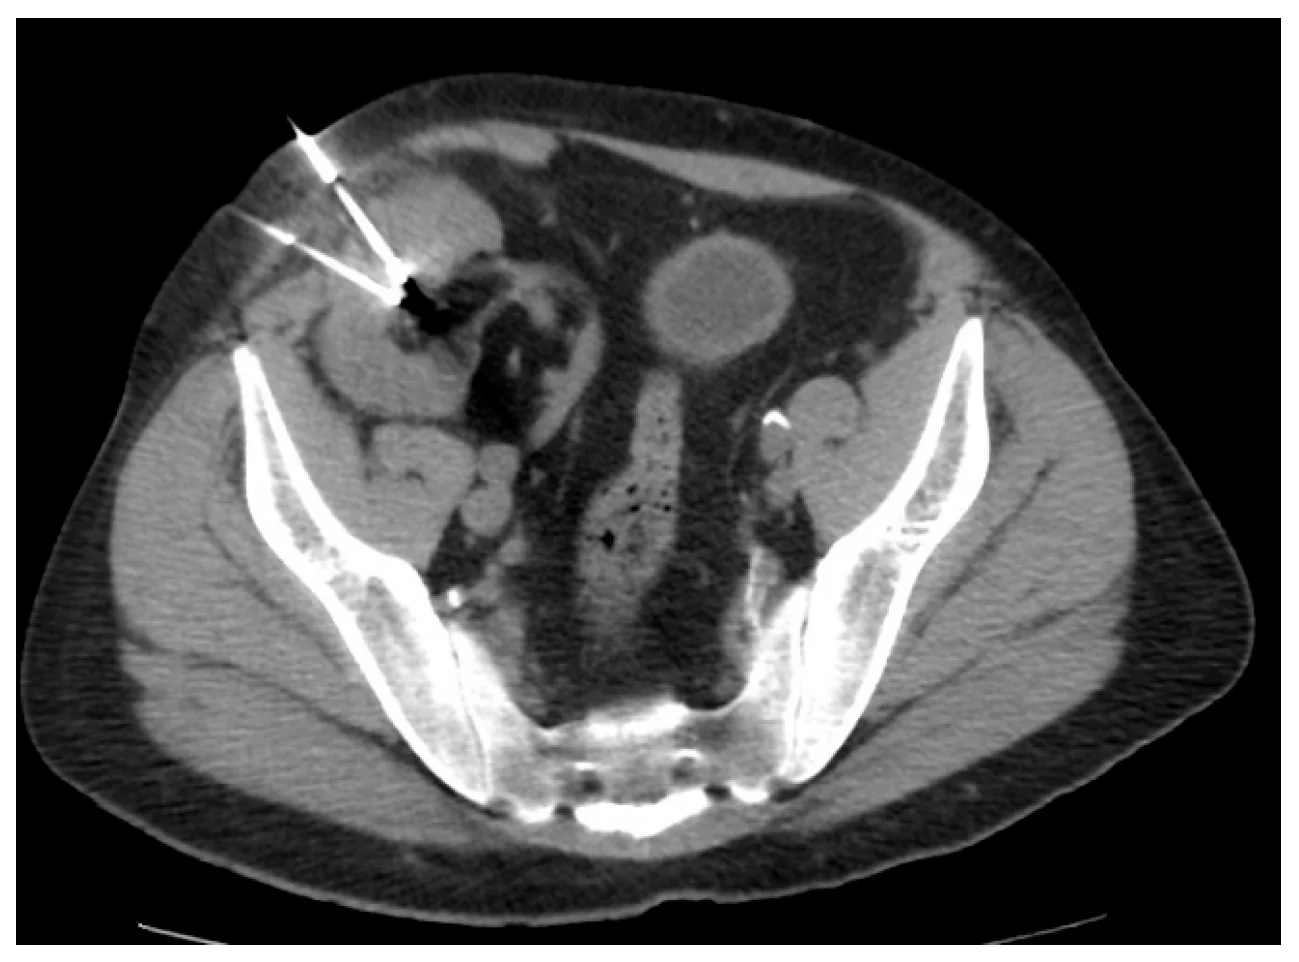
CT-guided percutaneous cryoablation of the renal allograft lesion. Intraprocedural CT image demonstrating cryoprobe placement within the tumor during cryoablation.
